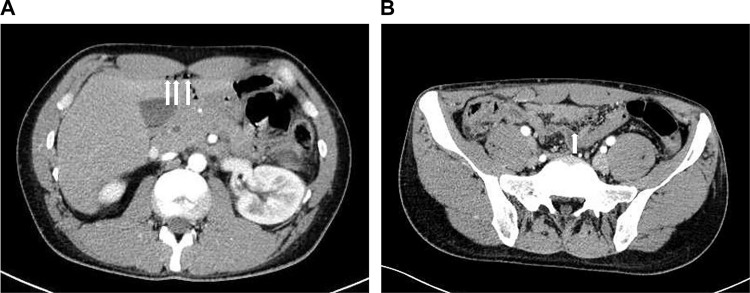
Pneumoperitoneum. (A) Axial section of CT of the abdomen showing free air on the surface of the liver (arrows). (B) Axial section of CT of the abdomen showing free air near the small intestine (arrows).
